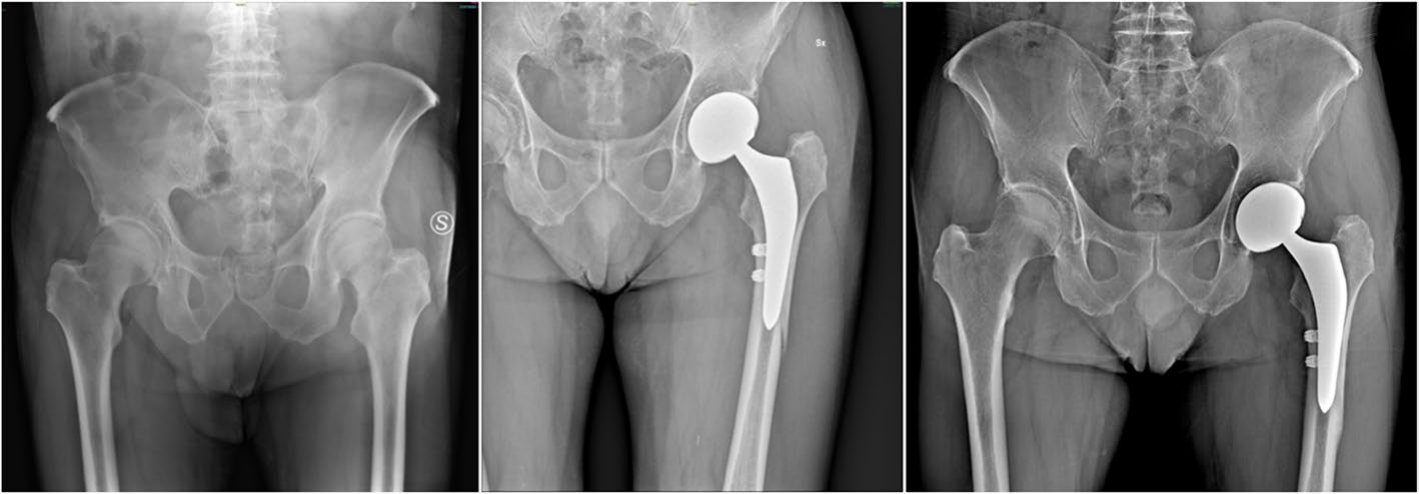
Pre and postoperative X-ray of a patient with femoral neck fracture undergoing total hip replacement through direct anterior approach. A periprosthetic femoral fracture was observed during the impaction of the stem and was managed by intraoperative wiring; at 12-month follow-up, complete fracture healing and bone remodeling were observed
